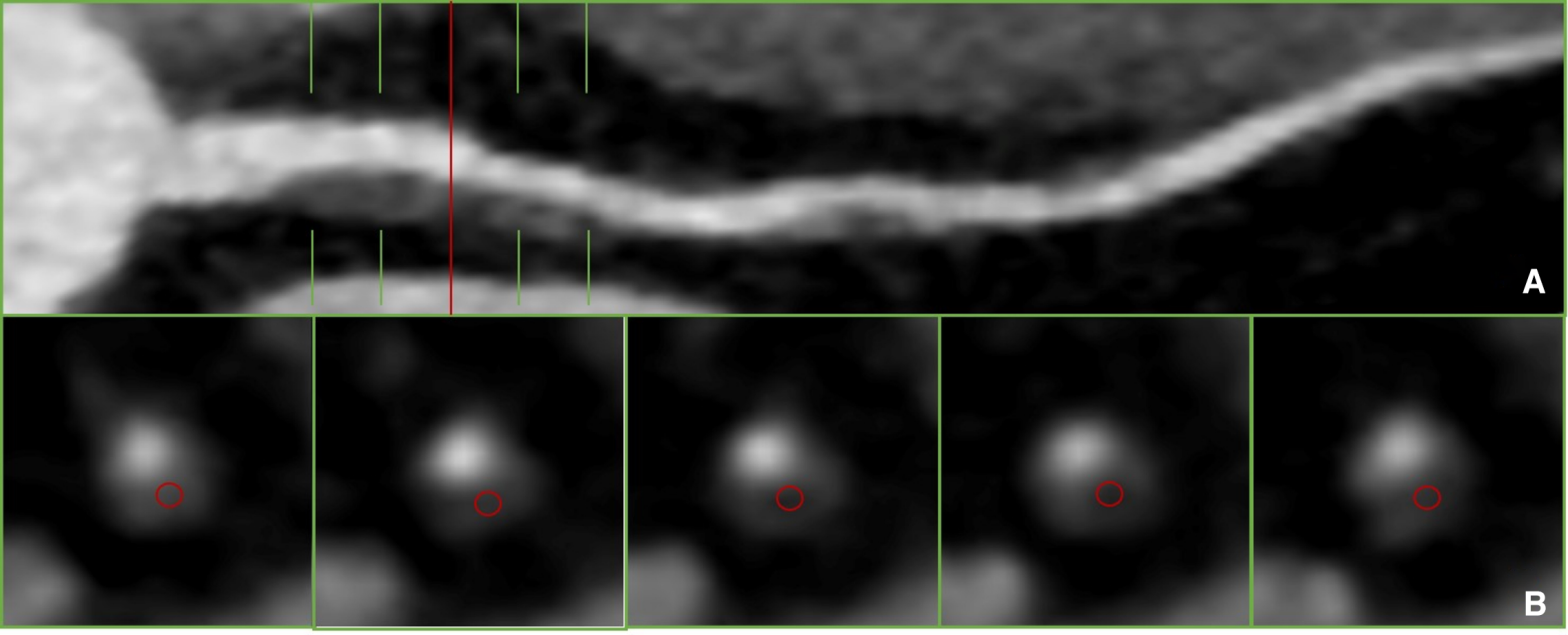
Plaque recognition and CT value measurement in previous literature (10). (A) Non-calcified plaque was detected on proximal segment of LAD on curved reformation. (B) The measurement of qualitative.
